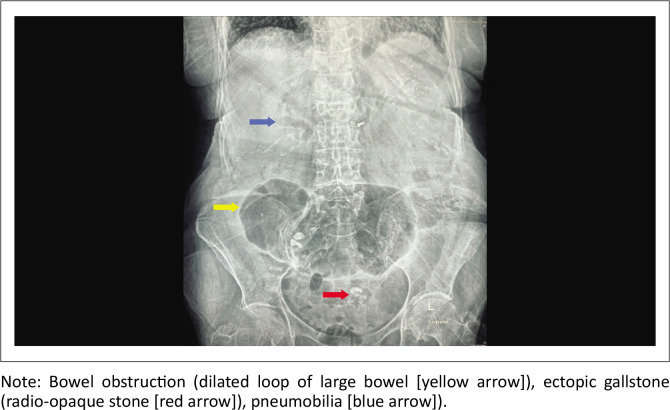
Plain supine abdominal radiograph demonstrating Rigler’s triad.
